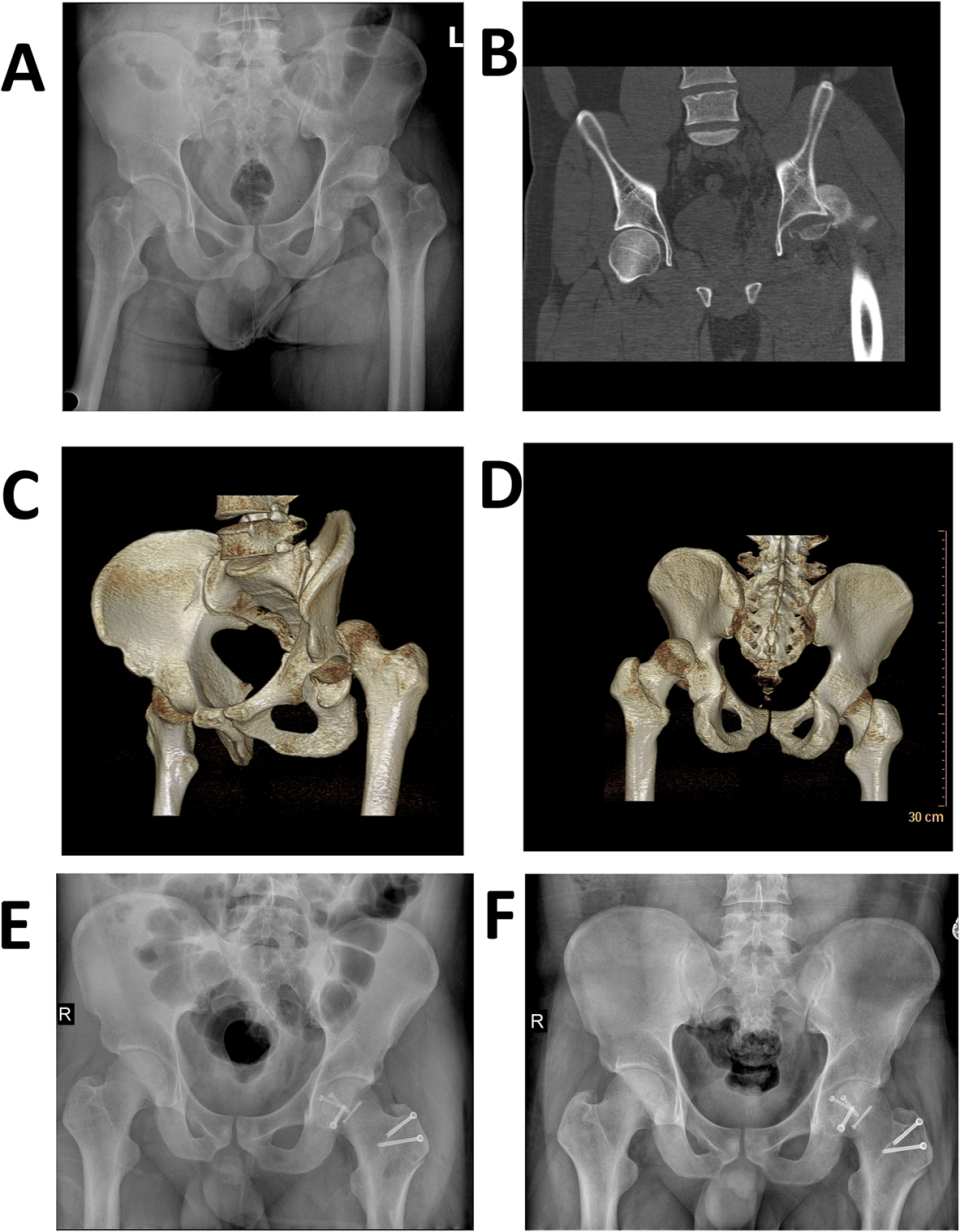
This was a 25-year-old male with femoral head fracture and hip dislocation by car accident. a The X-ray before operation. b–d The CT scan showed detail of the fracture. e The X-ray after operation. f the X-ray of 1 year after operation, which showed the fracture has healed and there was no AVN
